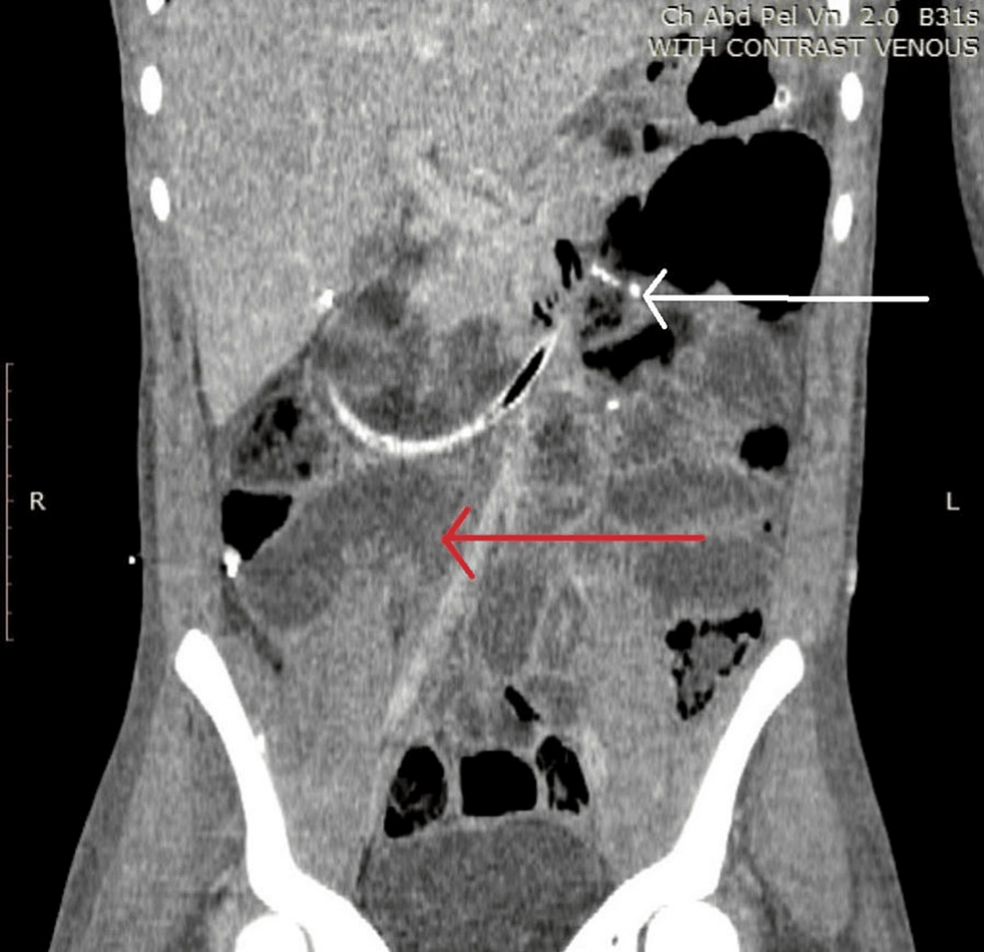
Coronal slice of the computed tomography scan with intravenous contrast in the venous phase showing dilated loops of the small bowel, with the ileocolic anastomotic staple line being seen in the left upper quadrant White arrow - ileocolic anastomotic staple line; red arrow - dilated loop of the small bowel
